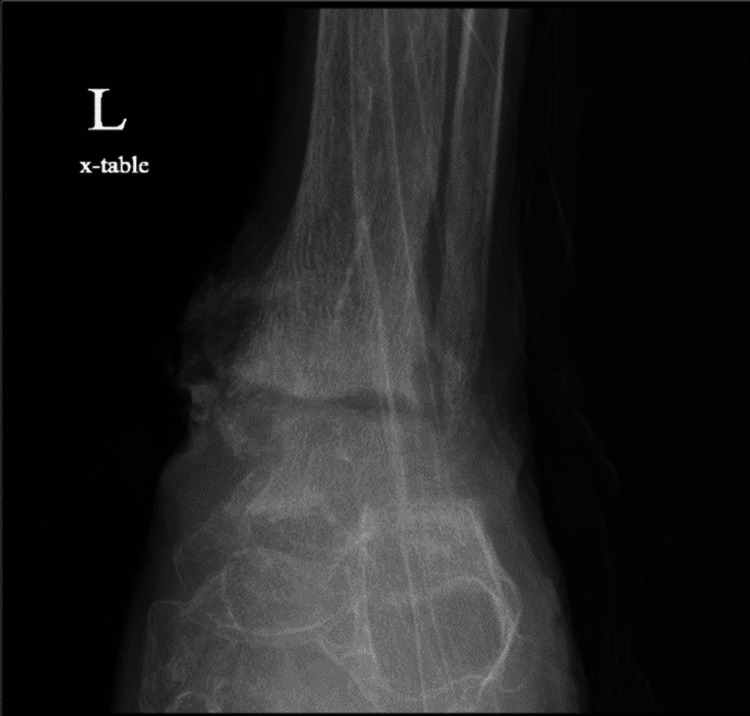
Anteroposterior X-ray of the left ankle/foot
